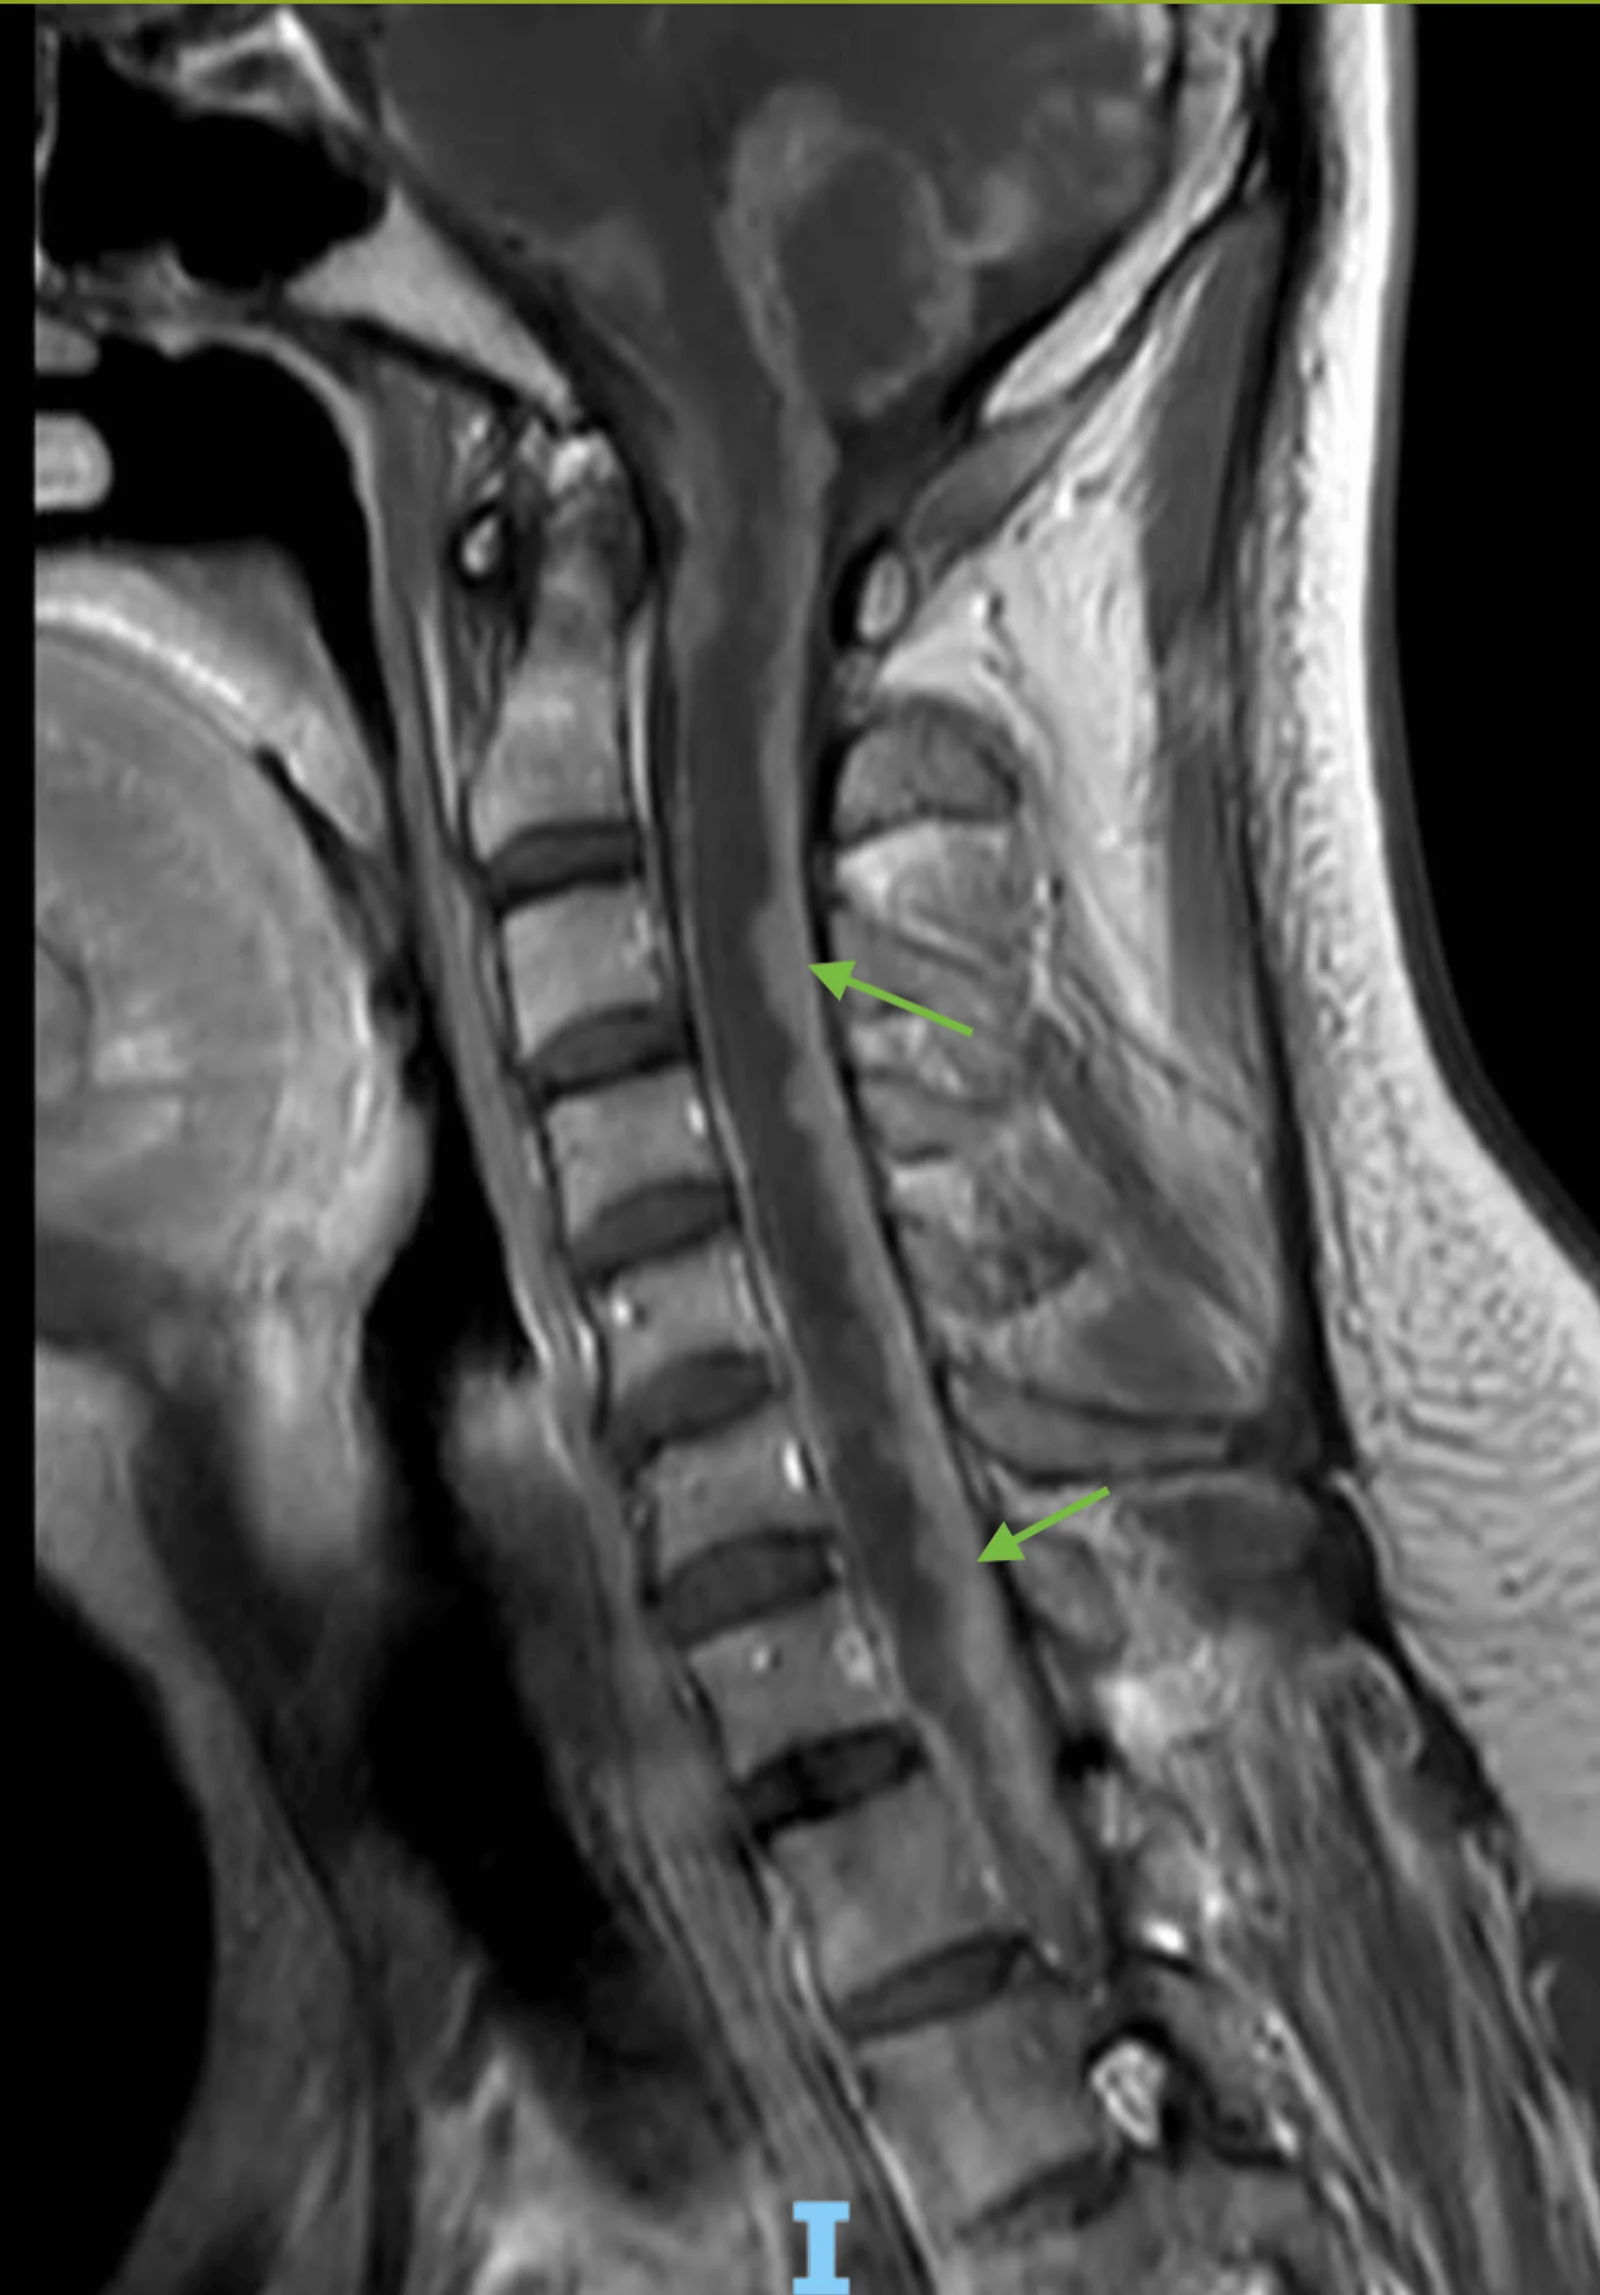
Cranial-cervical MRI, sagittal view with contrast medium. Diffuse cervical spinal arachnoiditis with nodular intradural enhancement along the spinal cord (green arrows).
